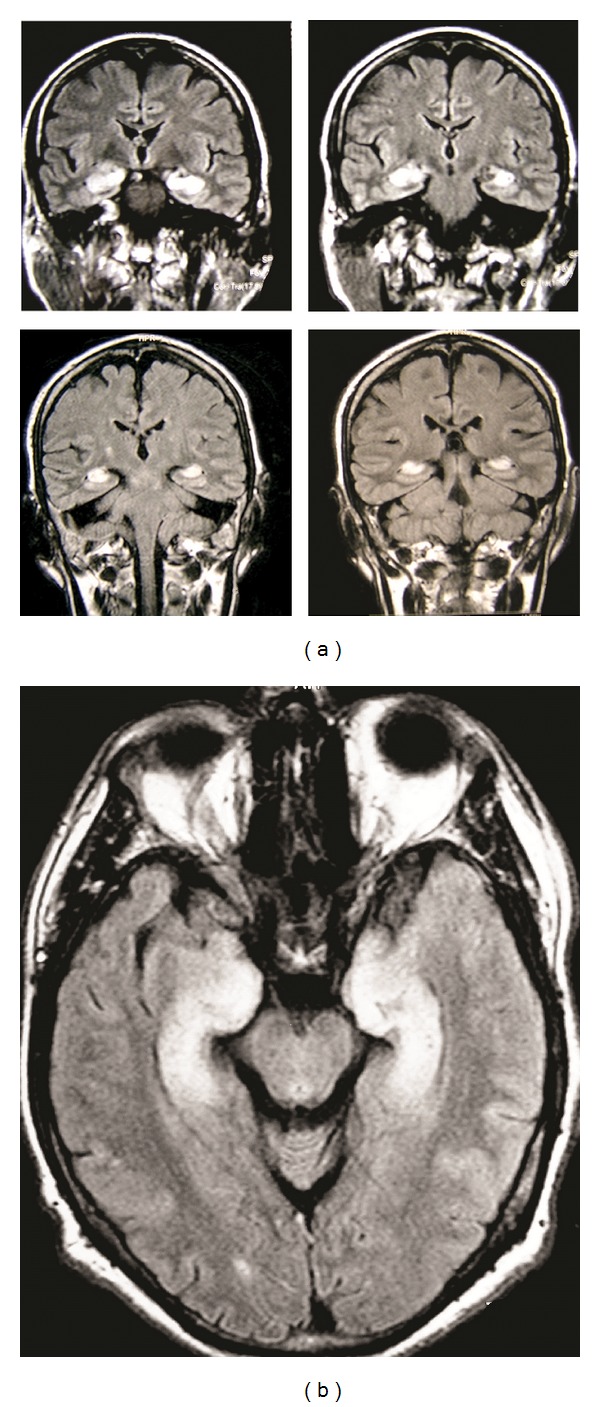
(a) Coronal FLAIR brain MR images demonstrate hyperintense lesions in medial temporal lobes bilaterally. (b) Axial FLAIR brain MR image demonstrates hyperintense lesions in medial temporal lobes bilaterally.
